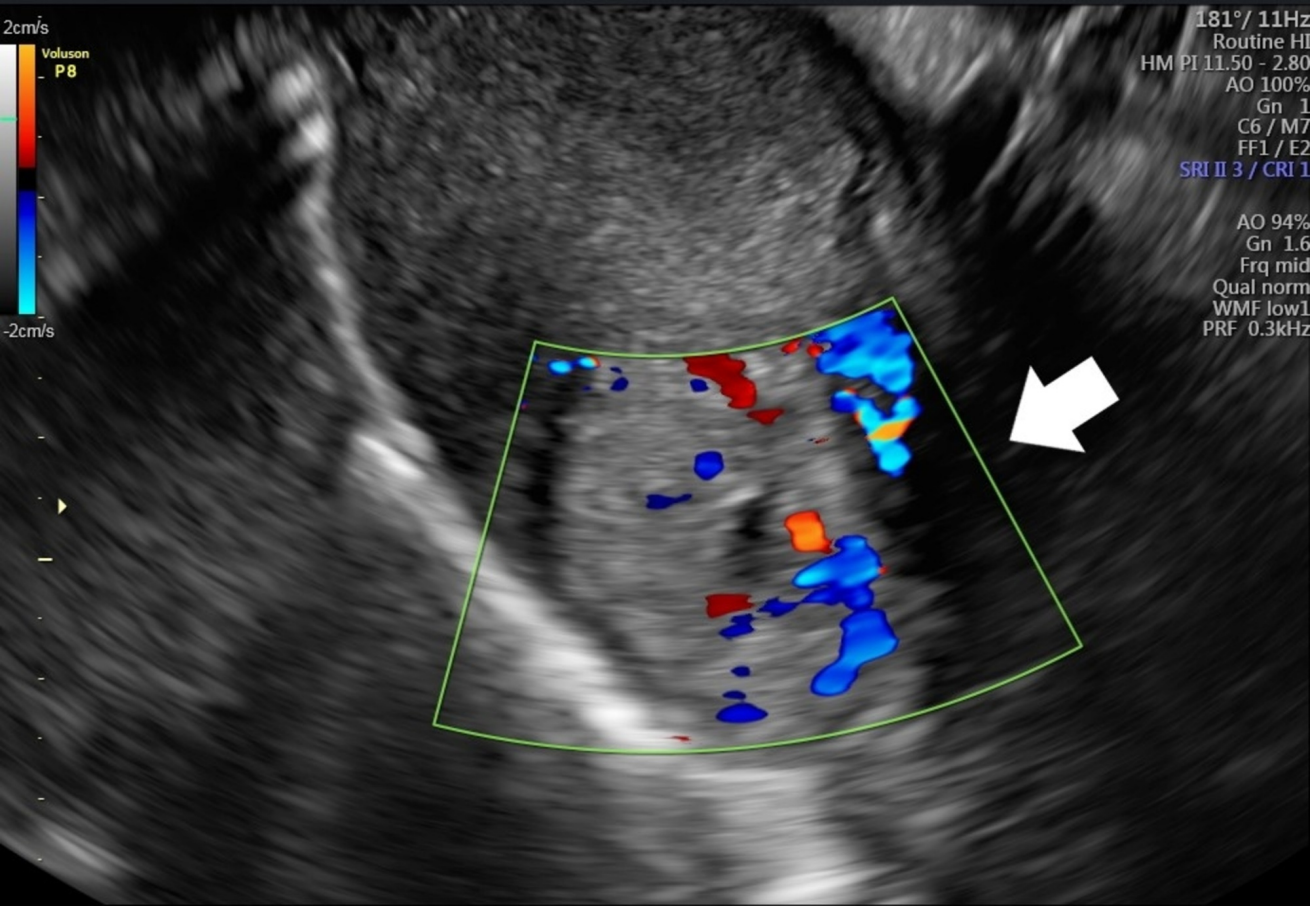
Doppler transvaginal ultrasound Doppler transvaginal ultrasound indicating echogenic structure in left cornual region (arrow).
